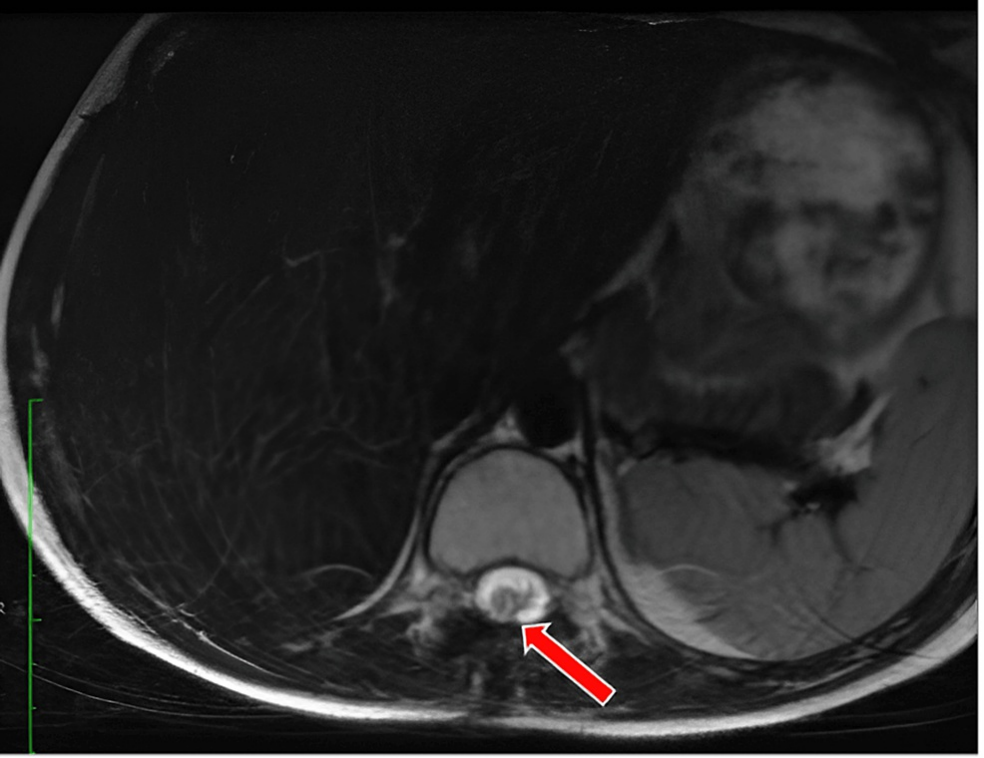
Preoperative MRI T2 sequence, lumbar spinal cord, axial section showing intramedullary lesion with infiltrative character
